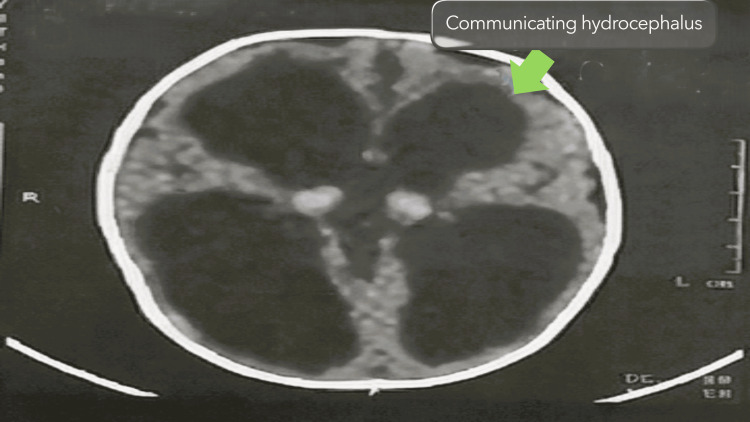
Postnatal CT brain showing communicating hydrocephalus
